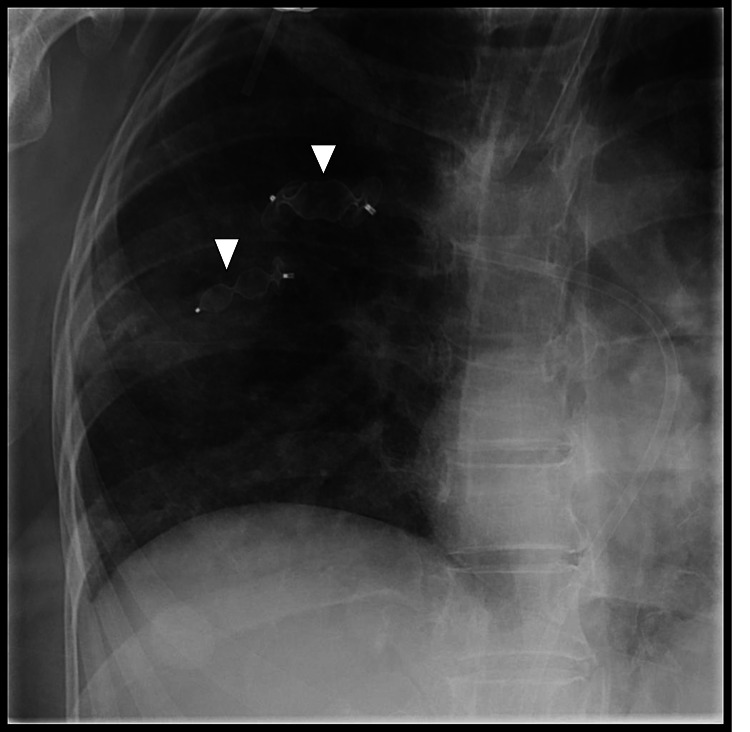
Plain radiograph image showing deployment of two Amplatzer vascular plugs (white arrowheads), one in each arterial feeders.
